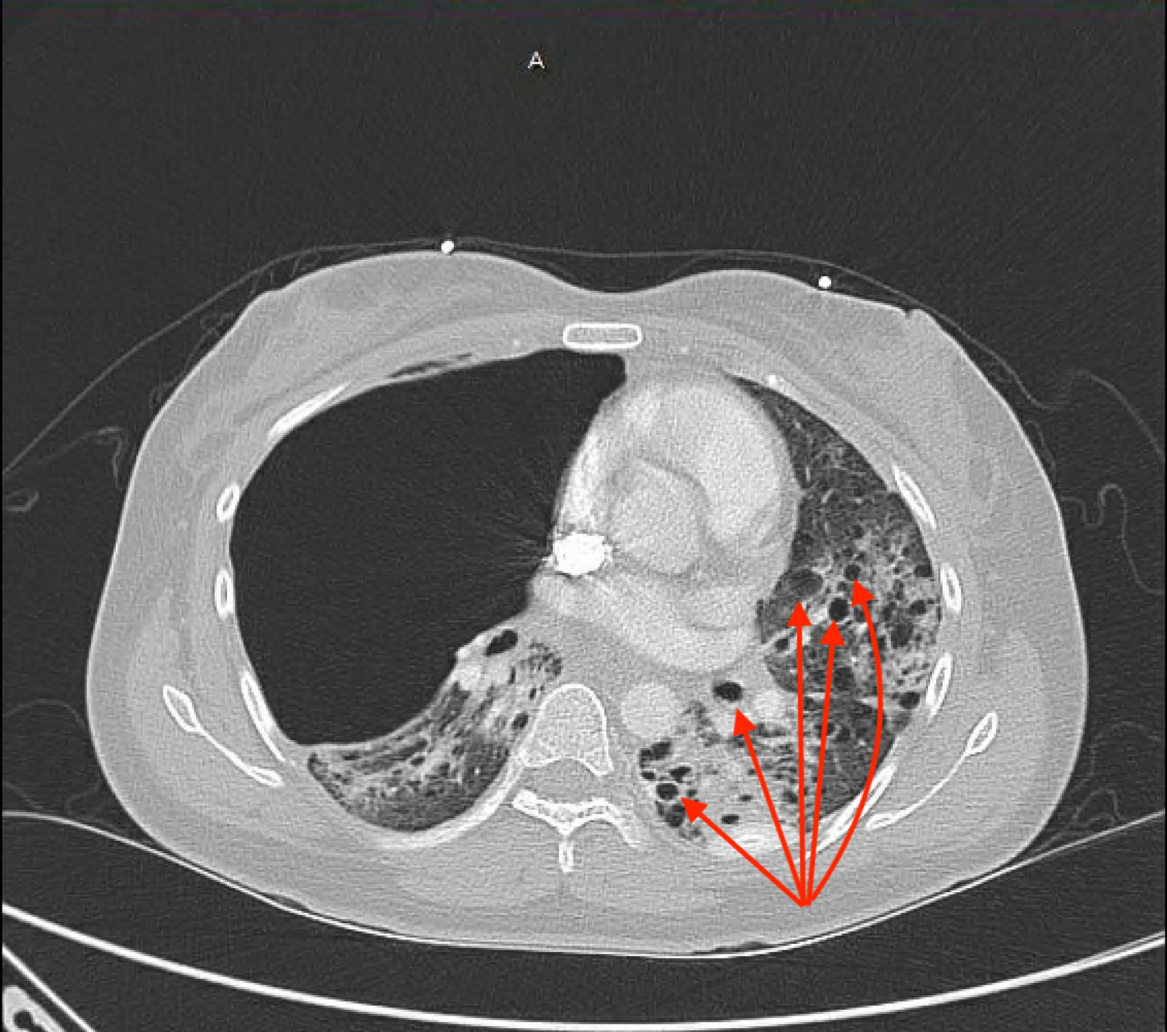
CT of the chest performed on follow-up visit showing multiple moderately thick-walled cysts located throughout the lung parenchyma with progression (red arrows).
